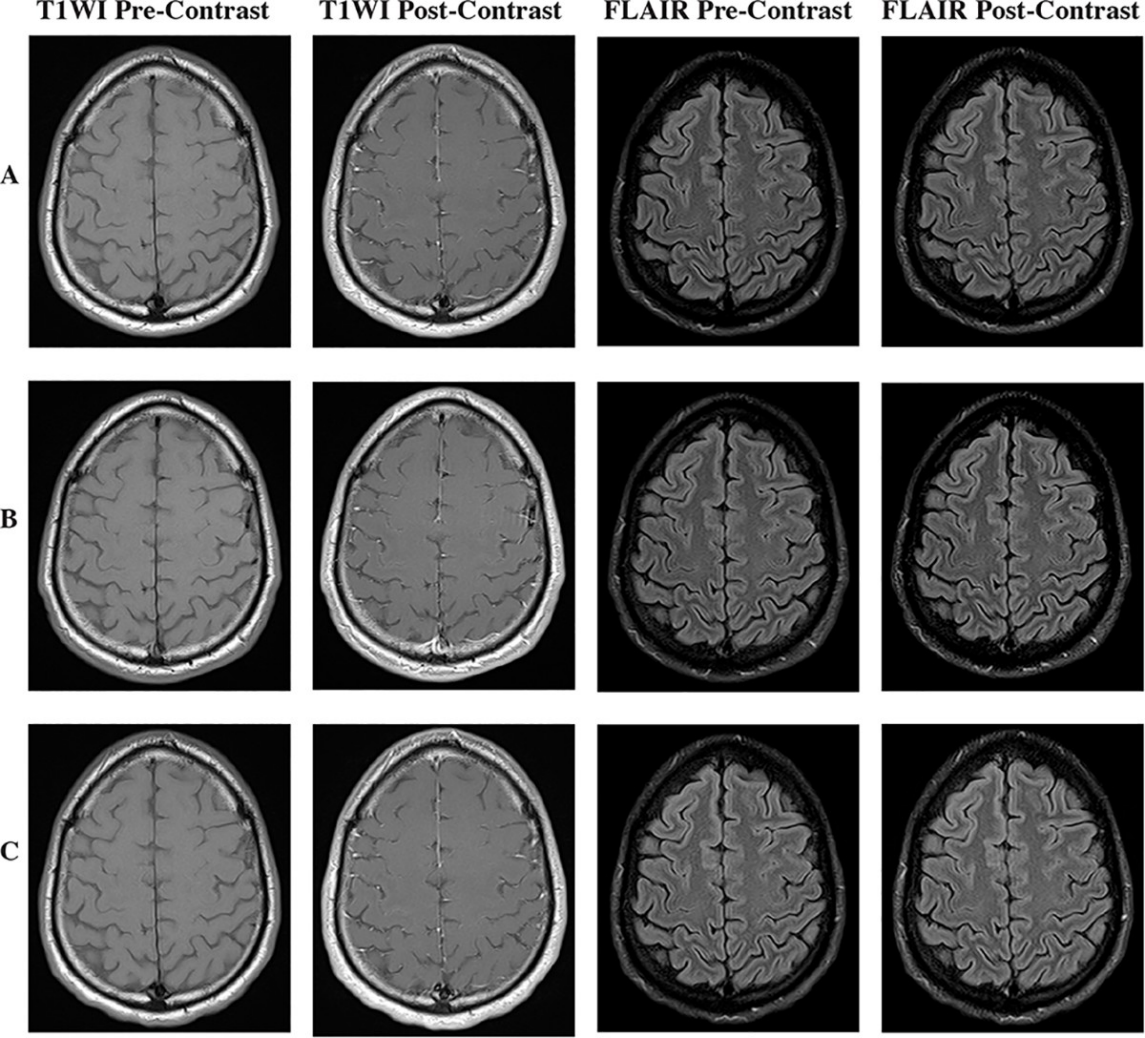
Imaging from the only subject scored positive for traumatic meningeal enhancement on T1WI post-contrast, but negative on FLAIR post-contrast. Pre-contrast T1WI and FLAIR images are shown for comparison. (A) According to raters this patient was scored positive due to the visualized pattern of contrast which seemed to thicken in the falx cerebri on the acute post-injury T1WI post-contrast sequences, while showing no findings on FLAIR post-contrast. (B) One week post-injury MRI shows a similar pattern of enhancement in falx cerebri-of T1WI post-contrast and a consistent lack of enhancement on FLAIR post-contrast. (C) T1WI and FLAIR post-contrast sequences one year post injury show once again a similar pattern of contrast on T1WI post-contrast sequence with no associated findings on FLAIR post-contrast sequence.
